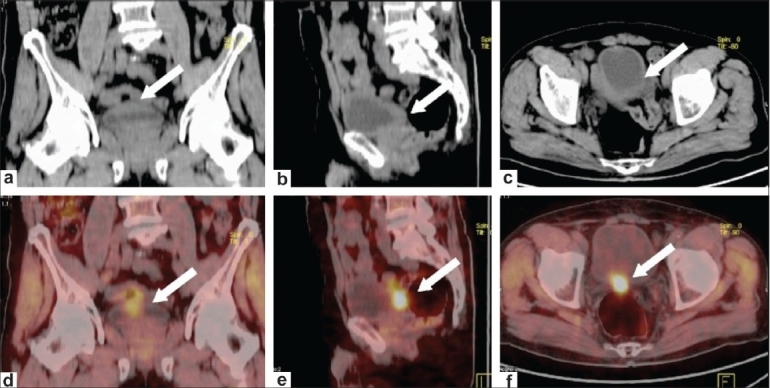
Postdiuretic delayed FDG-PET/CT acquisition in the same patient as in Figure 1. Coronal (a), sagittal (b) and axial (c) plain CT scan images reveal focal bladder wall thickening posteroinferiorly (arrows). Coronal (d), sagittal (e) and axial (f) fused PET/CT images reveal adequate tracer washout from the bladder. A focal area of hypermetabolism is noted in the thickened posteroinferior wall of the bladder (arrows), indicating active disease (recurrent cancer). Cystoscopic biopsy confirmed recurrence
